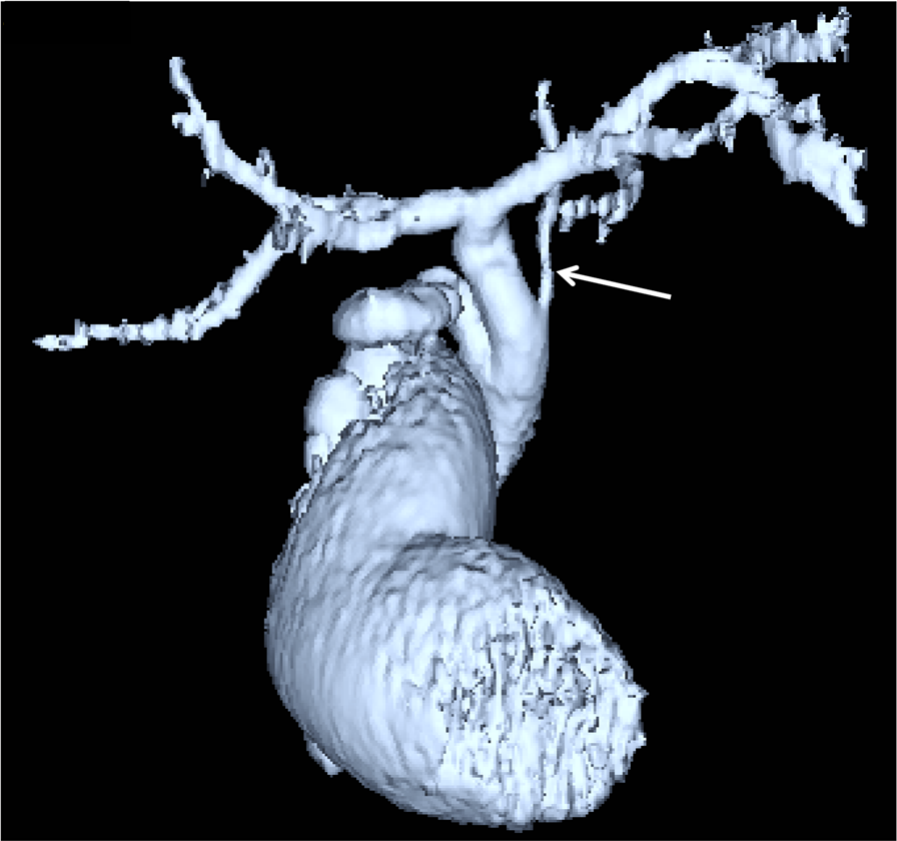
Three-dimensional DIC-CT image (ventral view) showing a type V accessory hepatic duct. The accessory hepatic duct drained the Spiegel lobe (*arrow*) and was classified into the caudate branch type
